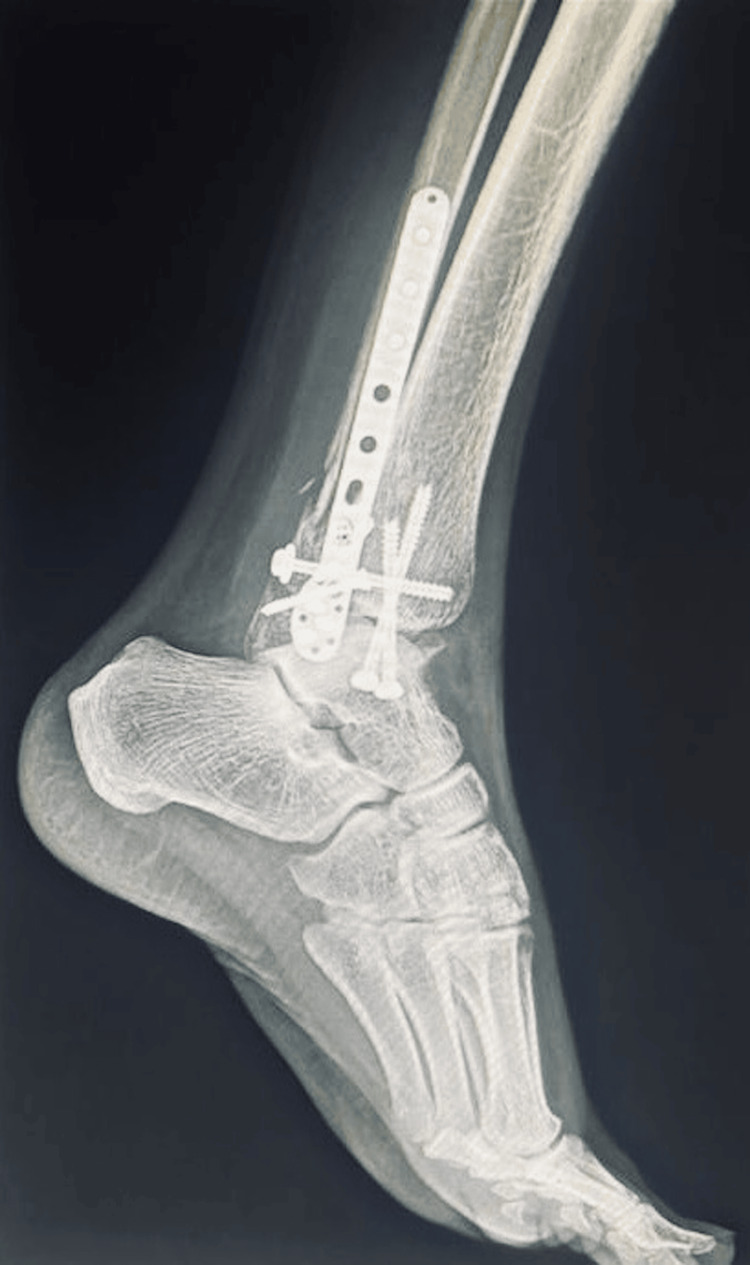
Post-operative X-ray (lateral view) of the left ankle.
